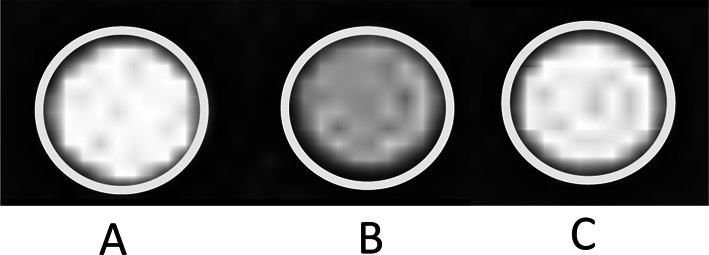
T1-weighted MR images of glass tubes containing Gadobenato dimeglumina (A), Mn(II)Cl_2_ × 4H_2_O (B), [Mn(II)(DASD)_2_] × 2H_2_O (C) in water at concentrations 3 mM obtained with 1.5 T a clinical Siemens Magnetom Aera (Reale et al. 2023). Original picture
